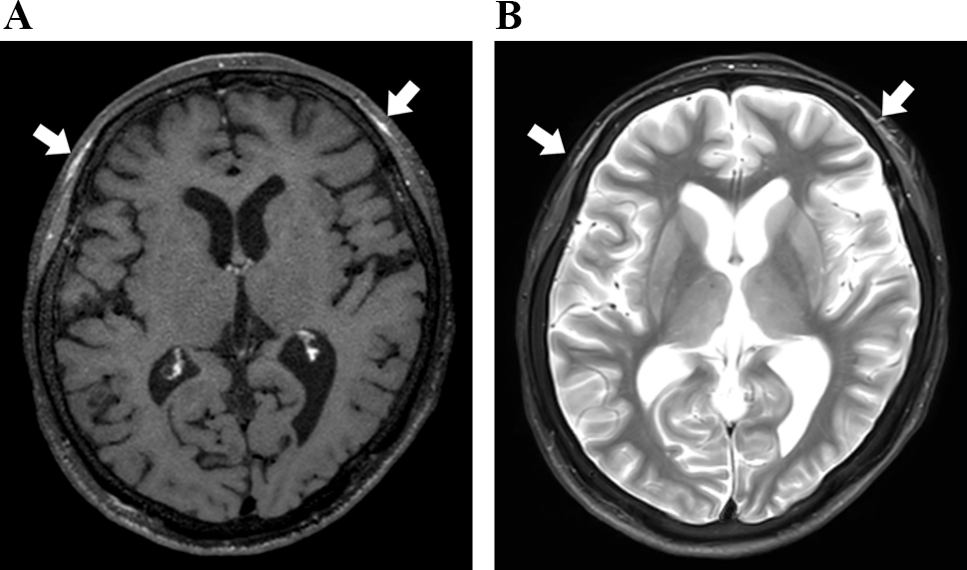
Case 16 (giant cell arteritis). (a), Contrast-enhanced T1-weighted (CE-T1W) image shows multiple distant arcuate enhancement areas of extracranial soft tissues. (b), STIR image shows high signal intensity areas of anterior extracranial soft tissues
